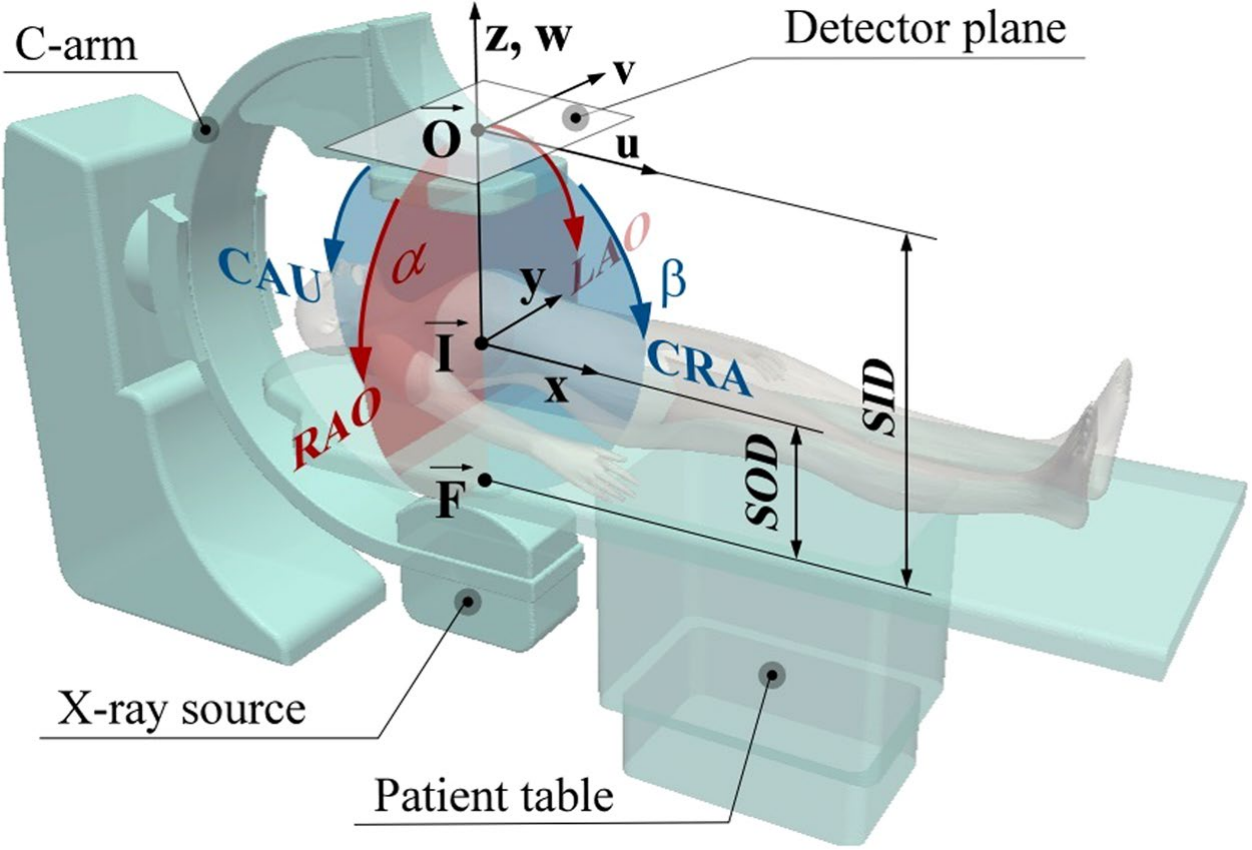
Construction of a conventional monoplane X-ray angiography device.
